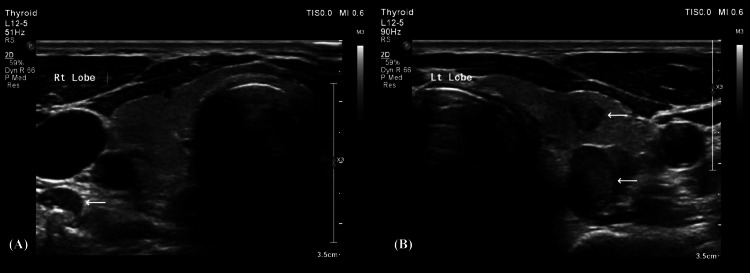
An ultrasound scan showing the right thyroid lobe (A) and the left thyroid lobe (B)
